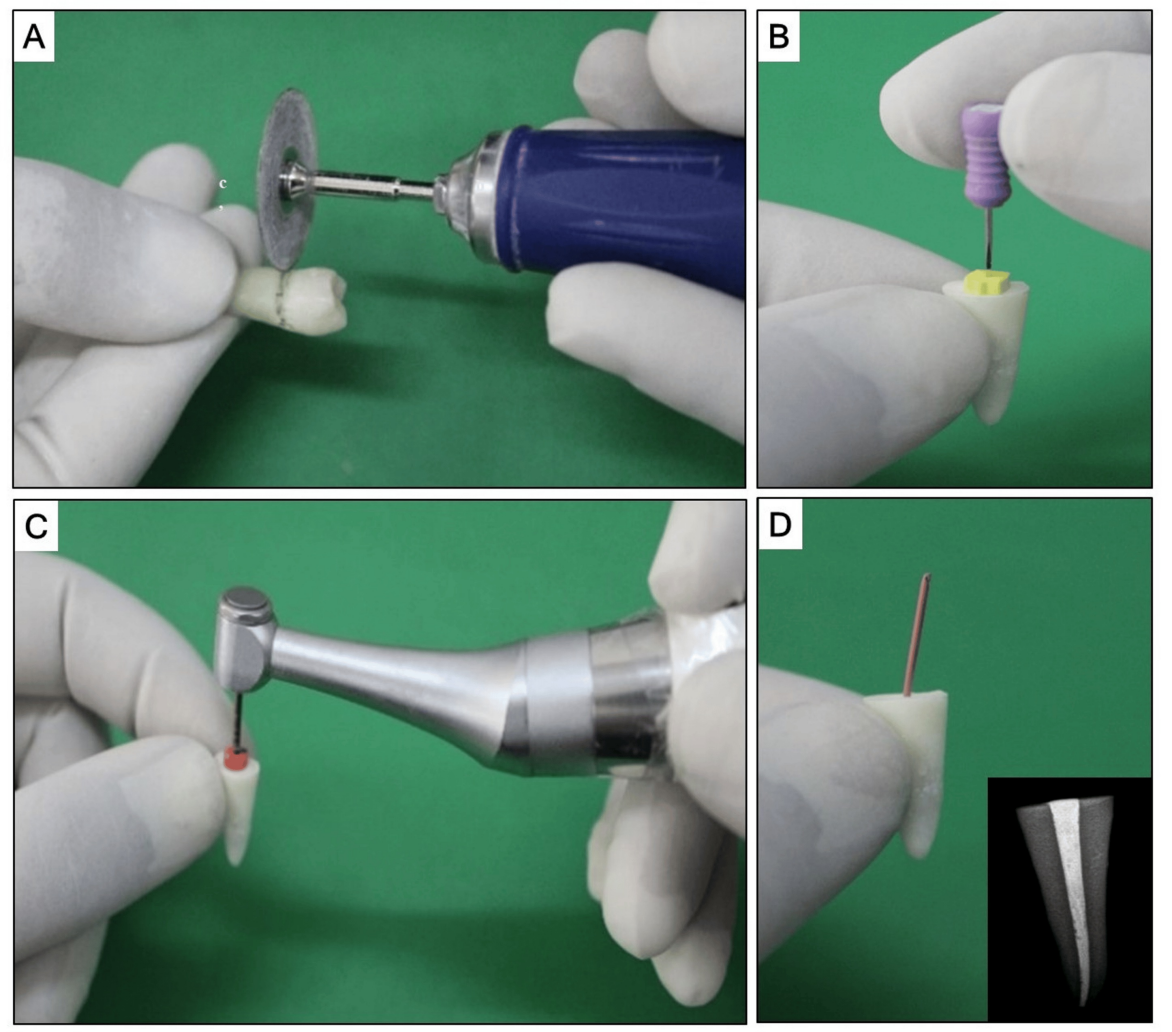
(A) Decoronation of the tooth at the CEJ level. (B) Determination of working length. (C) Preparation of the canals with Protaper rotary files. (D) Selection of appropriate master cone (inset: radiographic confirmation). CEJ, cementoenamel junction
